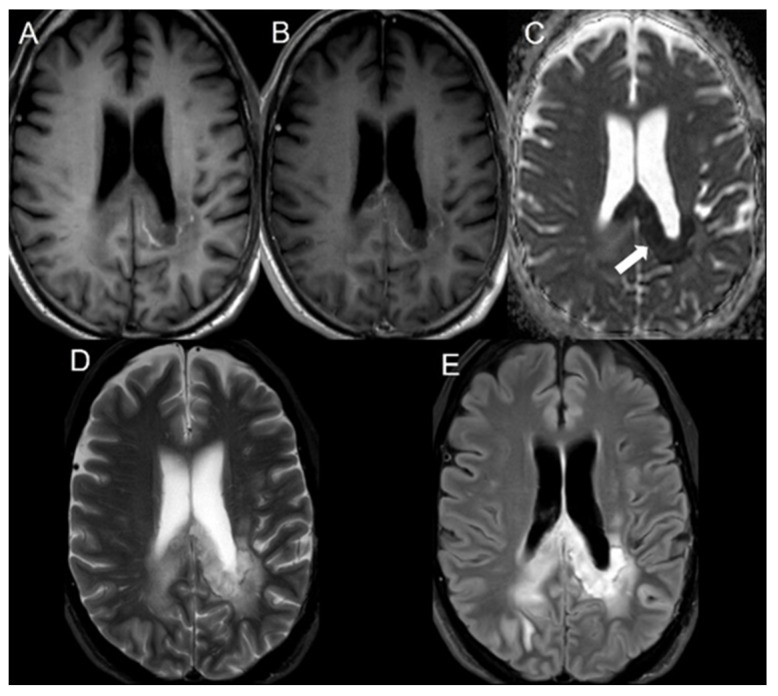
A 59 y/o male with bevacizumab-induced cytotoxicity. Axial MRI of the brain including (A) T1 weighted image, (B) postcontrast T1 weighted image, (C) ADC map, (D) T2 weighted image, and (E) FLAIR. The lesion demonstrates no contrast enhancement in the splenium of the corpus callosum (B) with restricted diffusion on the ADC map (white arrow in (C)). Mild adjacent areas of edema are seen in T2 weighted and FLAIR sequences (D,E).
